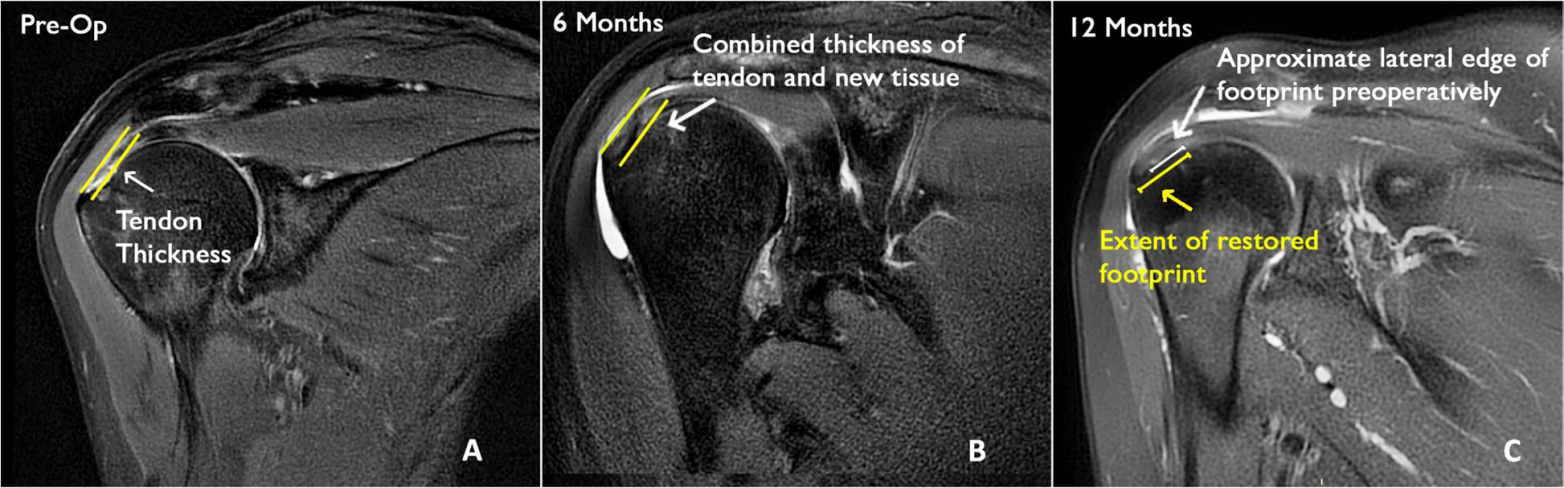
Coronal T2 weighted MRI image of a partial articular tear (A) and results after 6 (B) and 12 months (C), demonstrating the location (arrows) of the measurement of the combined thickness of the layer of new tissue and the underlying tendon
